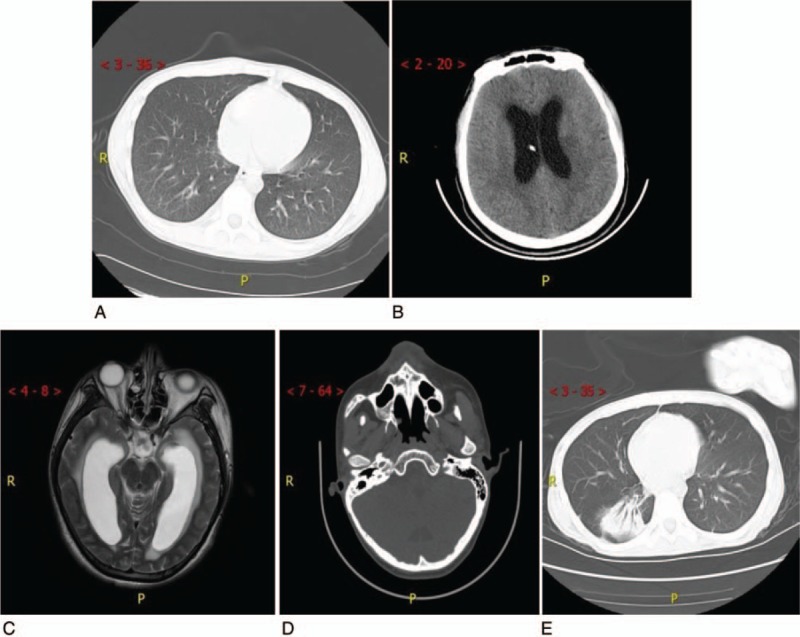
Computed tomography (CT) image after admission. (A) Nonenhanced pulmonary CT on Feb. 2, 2018. (B) Nonenhanced skull CT scan shows intracranial drainage with increased reduction of intracranial pressure. (C) Skull MRI upon first CSF culture shows XDRAB infection. (D) Nonenhanced skull CT scan after transfer to the rehabilitation unit. (E) Nonenhanced pulmonary CT scan after transfer to the rehabilitation unit. CSF = cerebrospinal fluid, CT = computed tomography, MRI = magnetic resonance imaging, XDRAB = extensively drug-resistant *Acinetobacter baumannii*.
